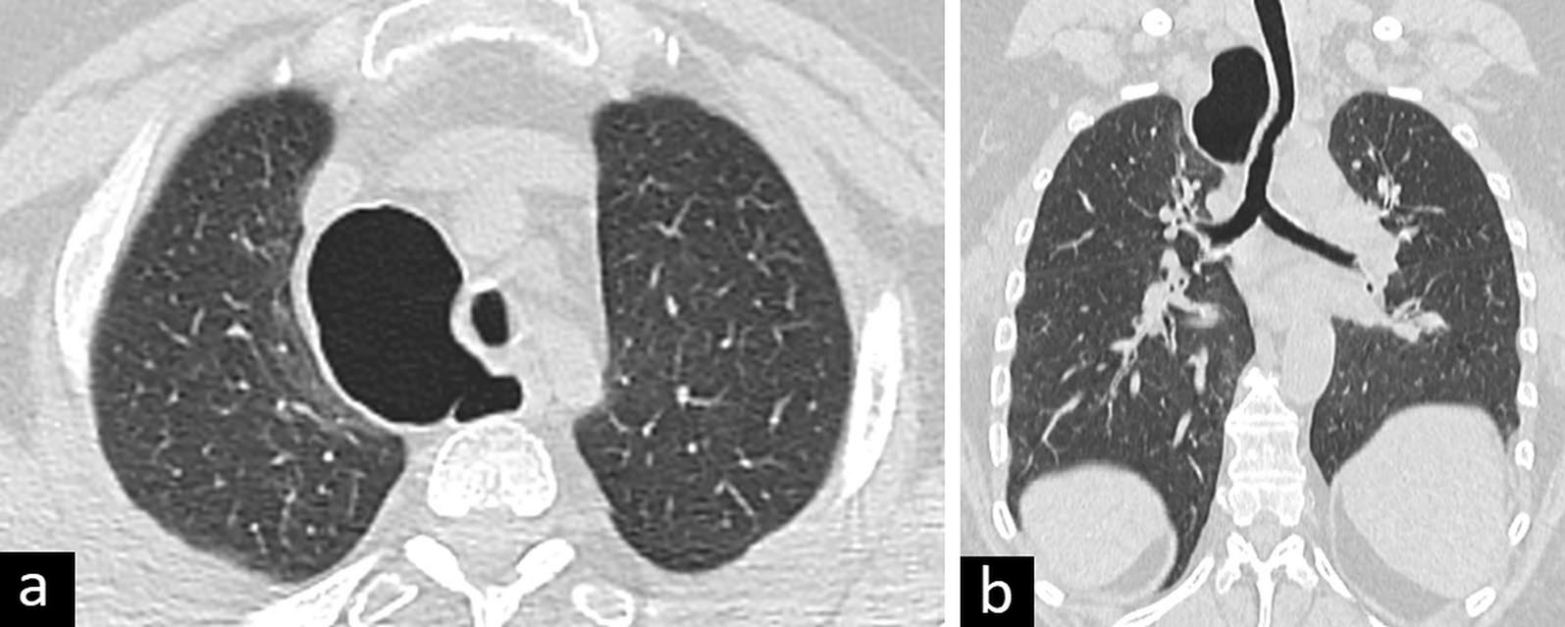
Tracheal diverticula in a 61-year-old woman presenting with dyspnea. Axial (a) and coronal (b) CT images demonstrate a giant right-sided paratracheal cyst
